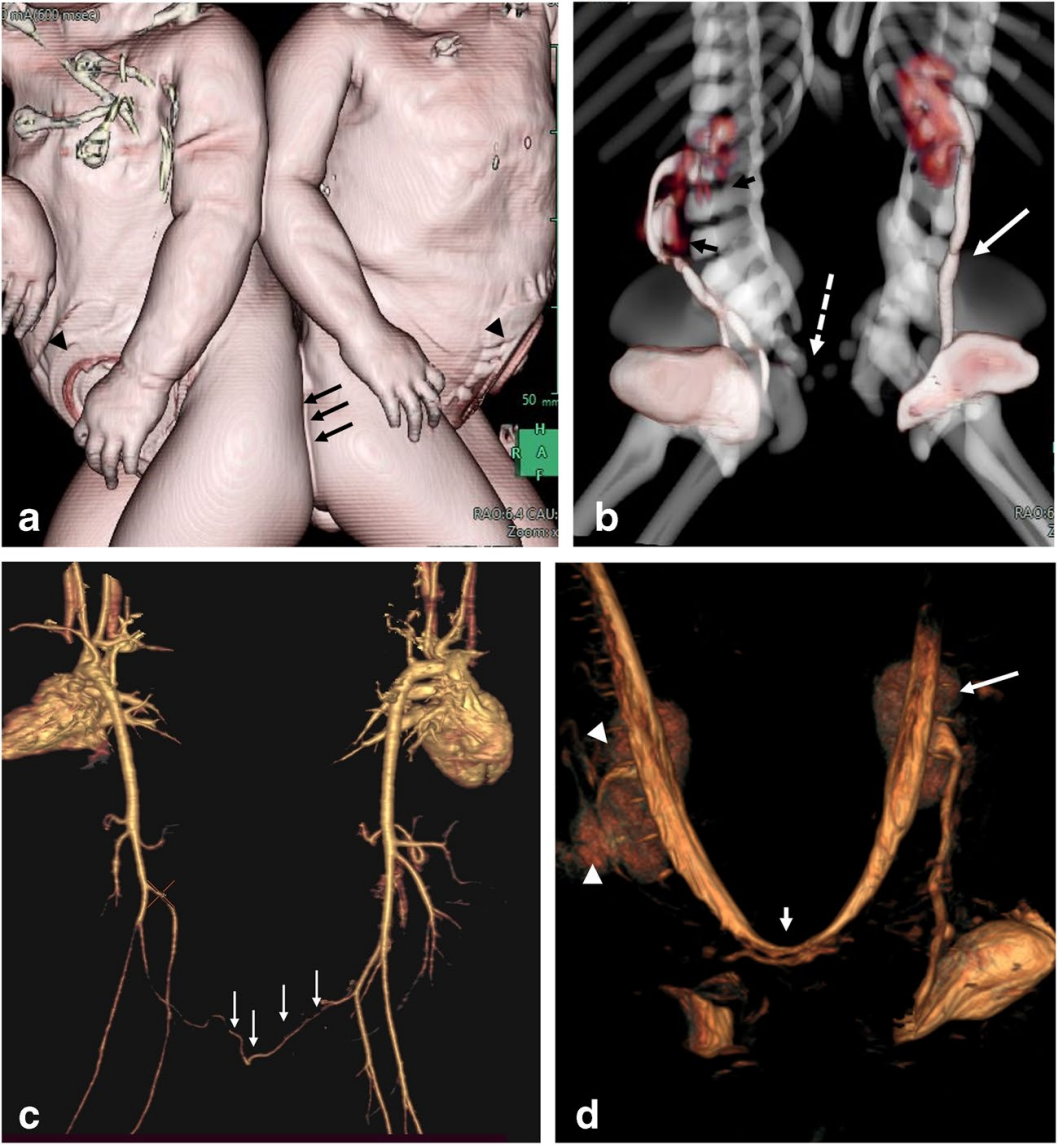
16-year-old pygopagus conjoined twin girls. a 3-dimensional (D) surface-rendered image reveals the site of fusion (*arrows*). Note the colostomy sites in both twins (*arrowheads*). b 3-D multimask image extracted from a computed tomography (CT) study in the excretory phase shows limited sacral fusion (*broken arrow*). The right twin has crossed fused kidneys (*black arrows*), while the left twin has a single kidney with crossed ectopia (*white arrow*). c CT angiography in the arterial phase (both twins injected simultaneously) shows arterial communication (*arrows*). d Maximum intensity projection of the coronal fat suppressed T2-weighted magnetic resonance image reveals that both thecal sacs are continuous through a narrow channel (*short arrow*). Note the single kidney of the left twin (*arrow*) and crossed fused ectopia of the right twin (*arrowheads*)
